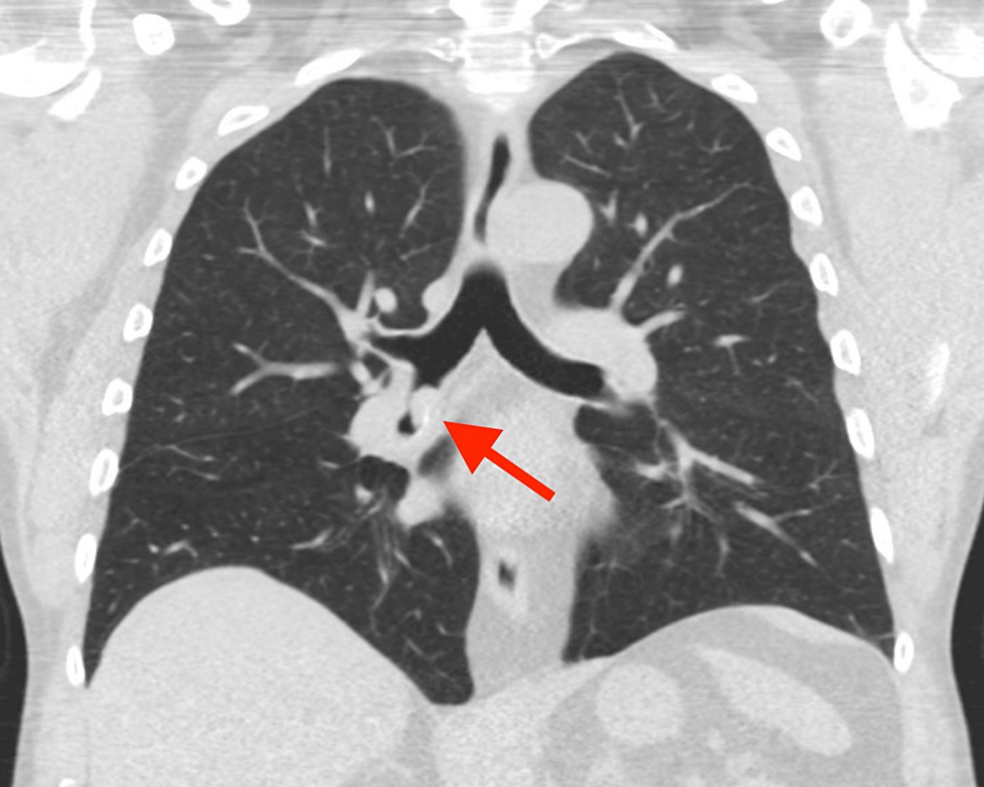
A coronal image of the same low-dose chest CT revealing the endobronchial lesion along the inferior aspect of the bronchus intermedius lumen (red arrow).
